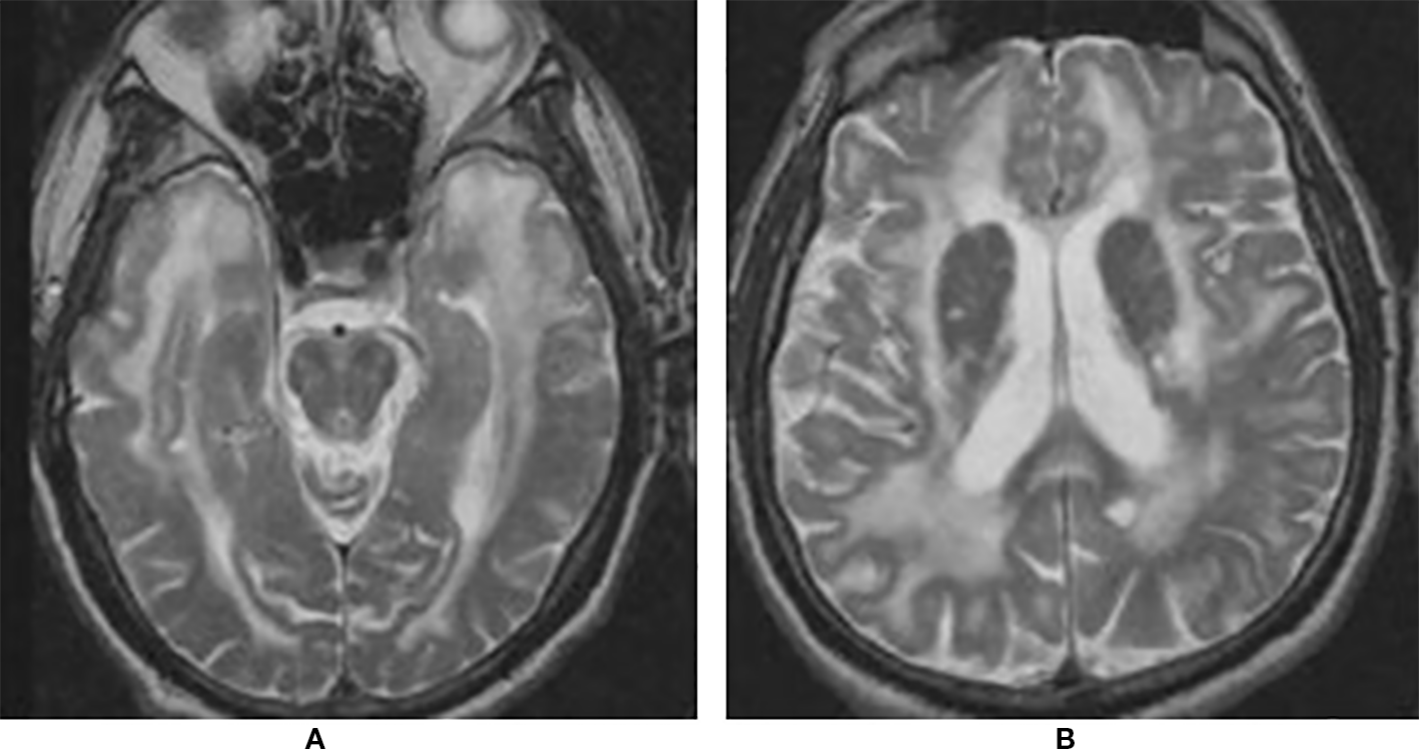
Brain magnetic resonance imaging (MRI) T2 showing extensive leukoencephalopathy with marked involvement of anterior temporal lobes (A) and external capsule (B).
